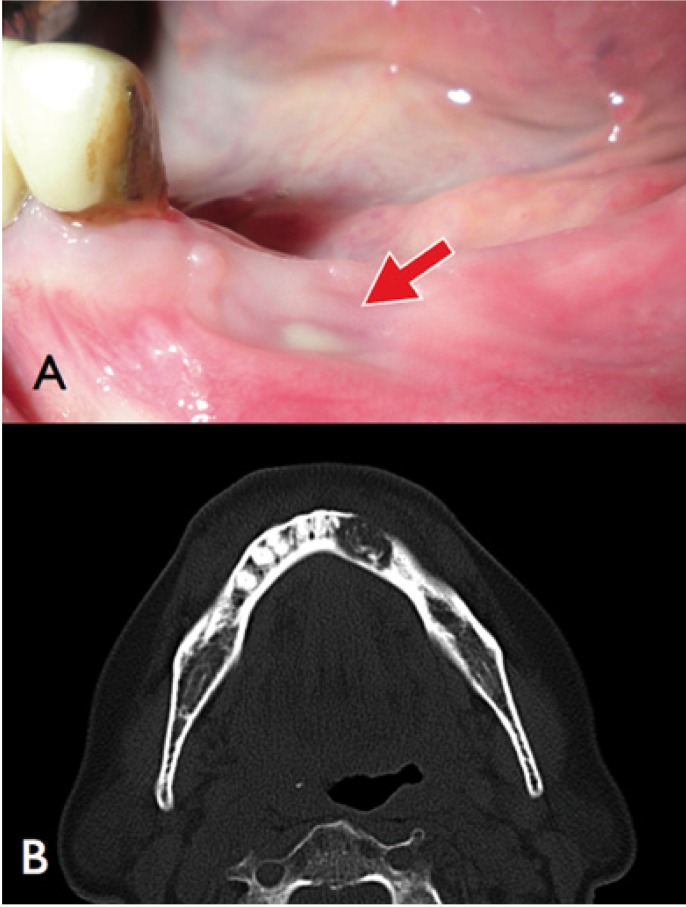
Case A4. Mandibular BRONJ with purulent sinus tract highlighted with an arrow (A). Computed tomography (B) shows the osteolytic pattern affecting the incisive mandibular area.
